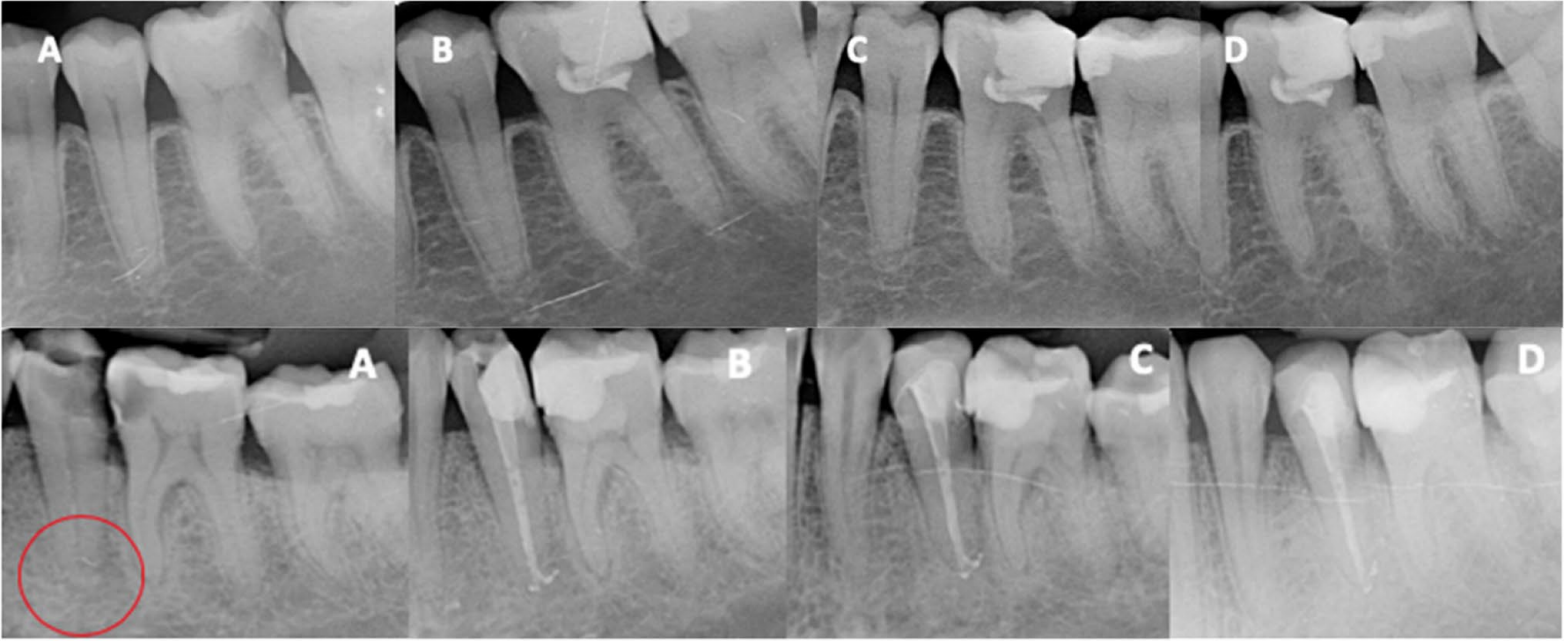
Top: full pulpotomy using Endosequence (A) Left lower first molar (36) with symptoms of irreversible pulpitis. (B) Full pulpotomy with Endosequence (this material is more radiopaque than biodentine). (C) Follow-up x-ray at 12 months. (D) Follow-up x-ray at 2-years. Bottom: full pulpotomy using Biodentine. (A) Left lower first molar (36) with symptoms of irreversible pulpitis and left lower second premolar (35) with pain on percussion, negative response to pulp test, and signs of apical periodontitis. (B) Full pulpotomy with Biodentine (36) and root canal treatment (35). (C) Follow-up x-ray at 12 months. (D) Follow-up x-ray at 2 years
